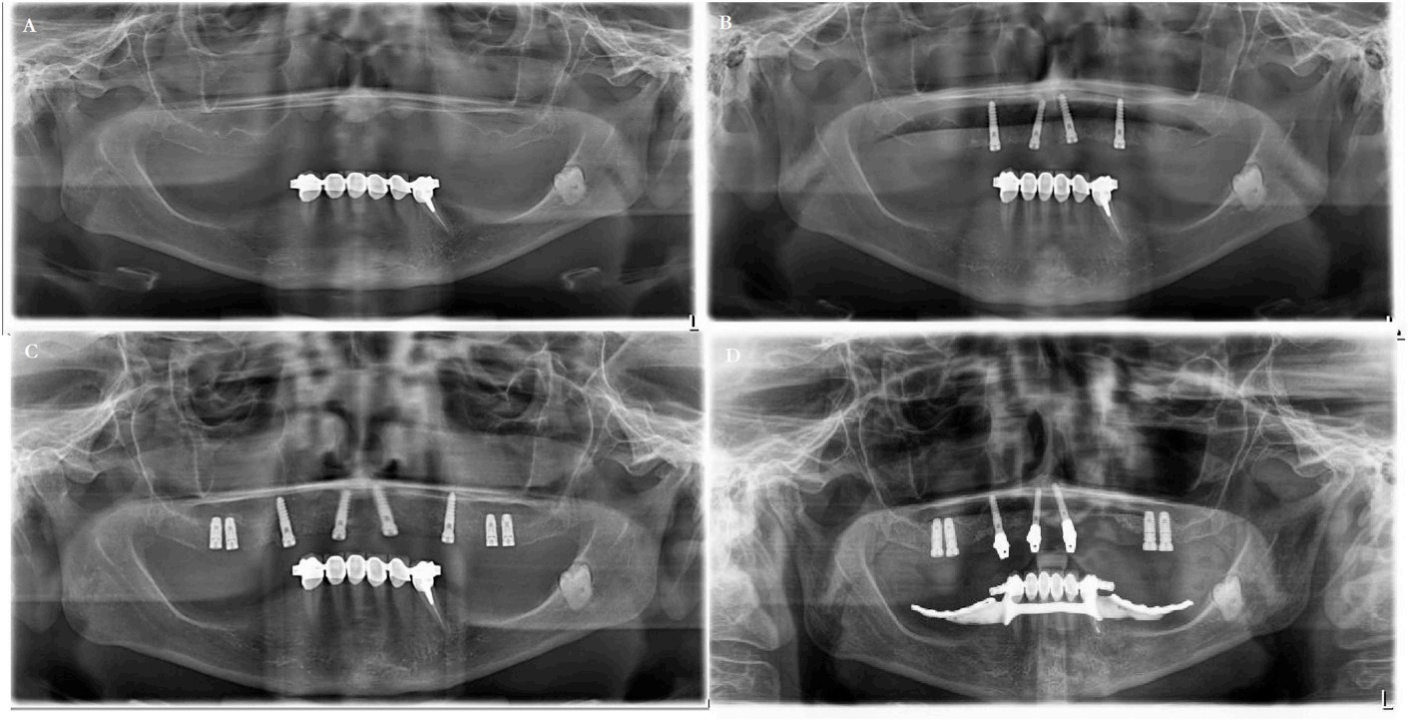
A,* re-surgical panoramic radiographic image (2014). *B,* Panoramic radiographic image demonstrating situation after first surgery (immediate implant treatment with four mini implants was performed ARST (2015). *C,* Panoramic radiographic image demonstrating situation after second surgery of implant placing in the molar region (2015). *D,* Panoramic radiographic image demonstrating in 2019.
